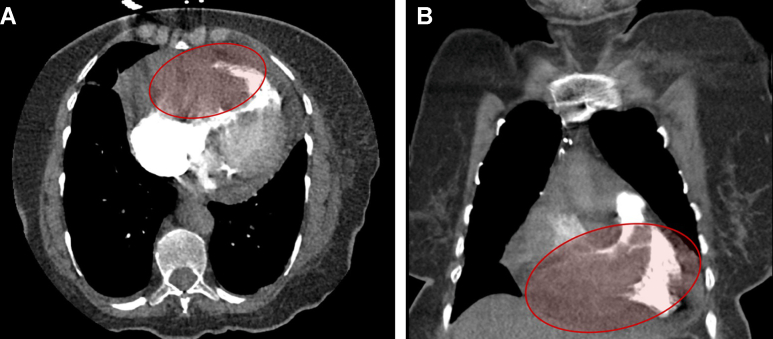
Contrast-Enhanced Chest CT Demonstrating Right Ventricular Mass (A and B) Axial view (top) and coronal view (bottom) of contrast-enhanced CT of the chest demonstrating a mass within the right ventricle (RV), extending toward the RV outflow tract. The mass appears hyperdense relative to the surrounding myocardium, suggesting a solid neoplastic process rather than a thrombus. A small pericardial effusion is noted, without significant mediastinal lymphadenopathy.
